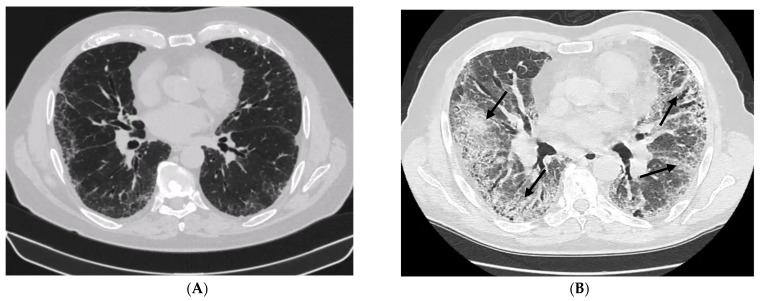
(A) HRCT of an IPF patient at baseline, showing a UIP pattern. (B) HRCT of an IPF patient with bilateral GGO and consolidations superimposed on the UIP pattern corresponding to the clinical diagnosis of AE-IPF. Arrows indicate newly developed consolidations and GGOs superimposed on a UIP pattern.
